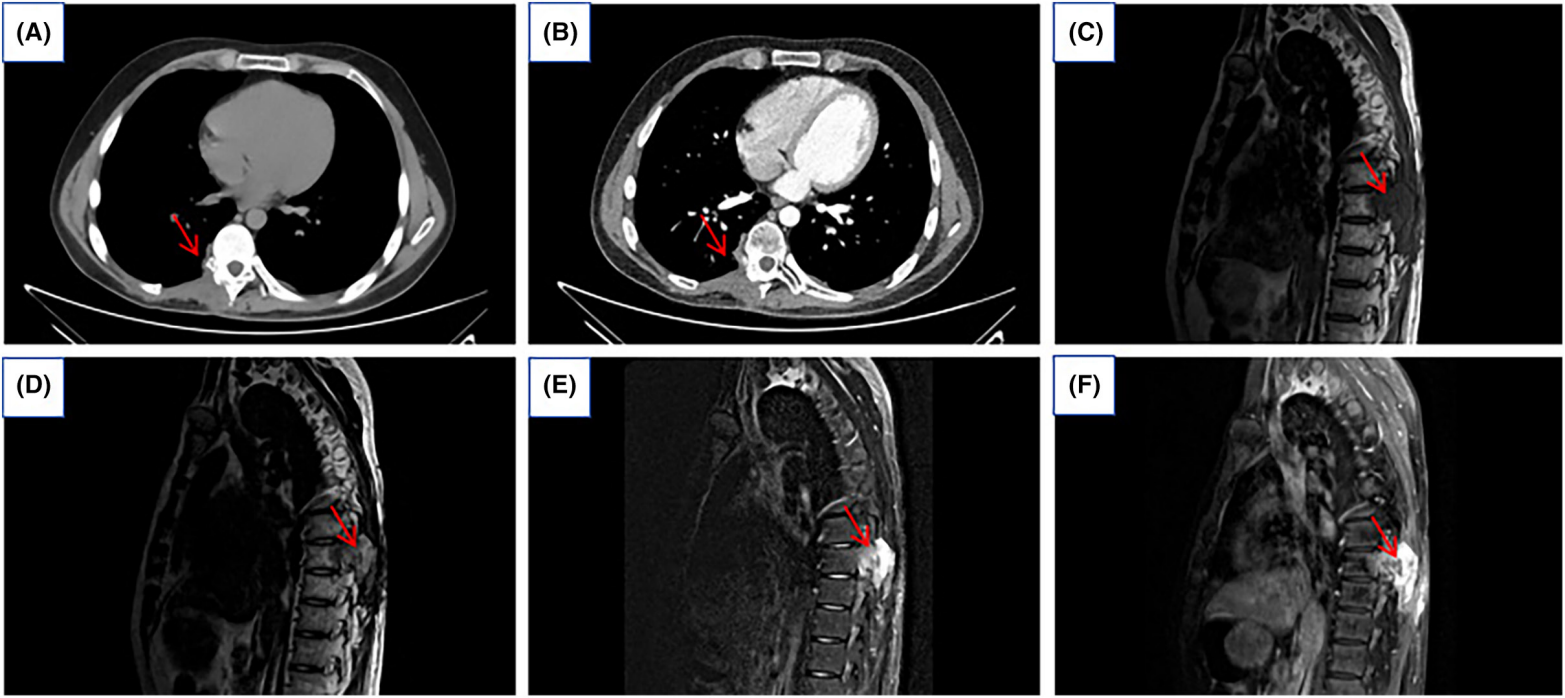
Six months postoperative follow‐up: (A) CT plain scan showed that some of the right‐sided arch boards of thoracic 8 and 9 and the right‐sided transverse processes, localized bony defects of the right‐sided 8th and 9th ribs show postoperative changes, and soft tissue thickening of the right‐sided trapezius and erector spinae muscles; (B) CT enhancement scan showed no abnormal enhancement (red arrows in A and B). Twelve months postoperative follow‐up: MRI showed a right paraspinal mass‐like mixed‐signal shadow at the level of thoracic 8 and 9 vertebrae; (C) T1WI sagittal lesion was isosinusoidal; (D) T2WI sagittal was isosinusoidal with a predominantly slightly higher signal; (E) T2WI sagittal compression lipids showed predominantly high signal with isohyperintense signal, and the lesion was poorly demarcated from the right thoracic erector spinae muscle. (F) T1WI sagittal enhancement scans of the lesion showed marked inhomogeneous enhancement, with small strips of unstrengthened areas within it, and the boundaries were poorly defined (red arrows in C, D, E, and F).
